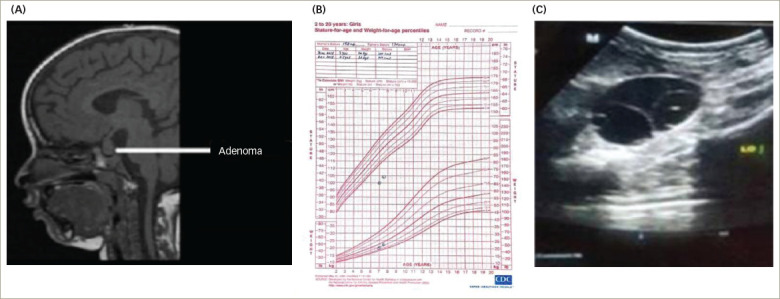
Endocrine Work-up Case 1. A: Pituitary MRI showing thyrotrope hyperplasia mimicking a pituitary adenoma; B: Growth chart; C: Abdominal ultrasound showing polycystic ovaries
